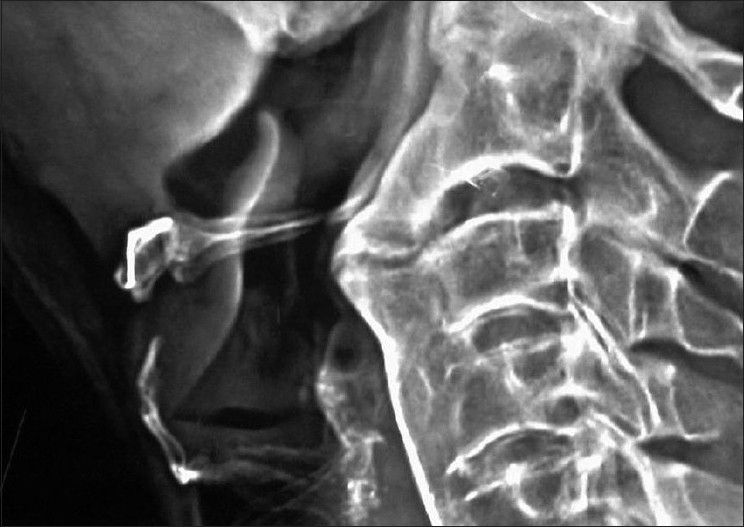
Lateral x-ray of cervical spine
